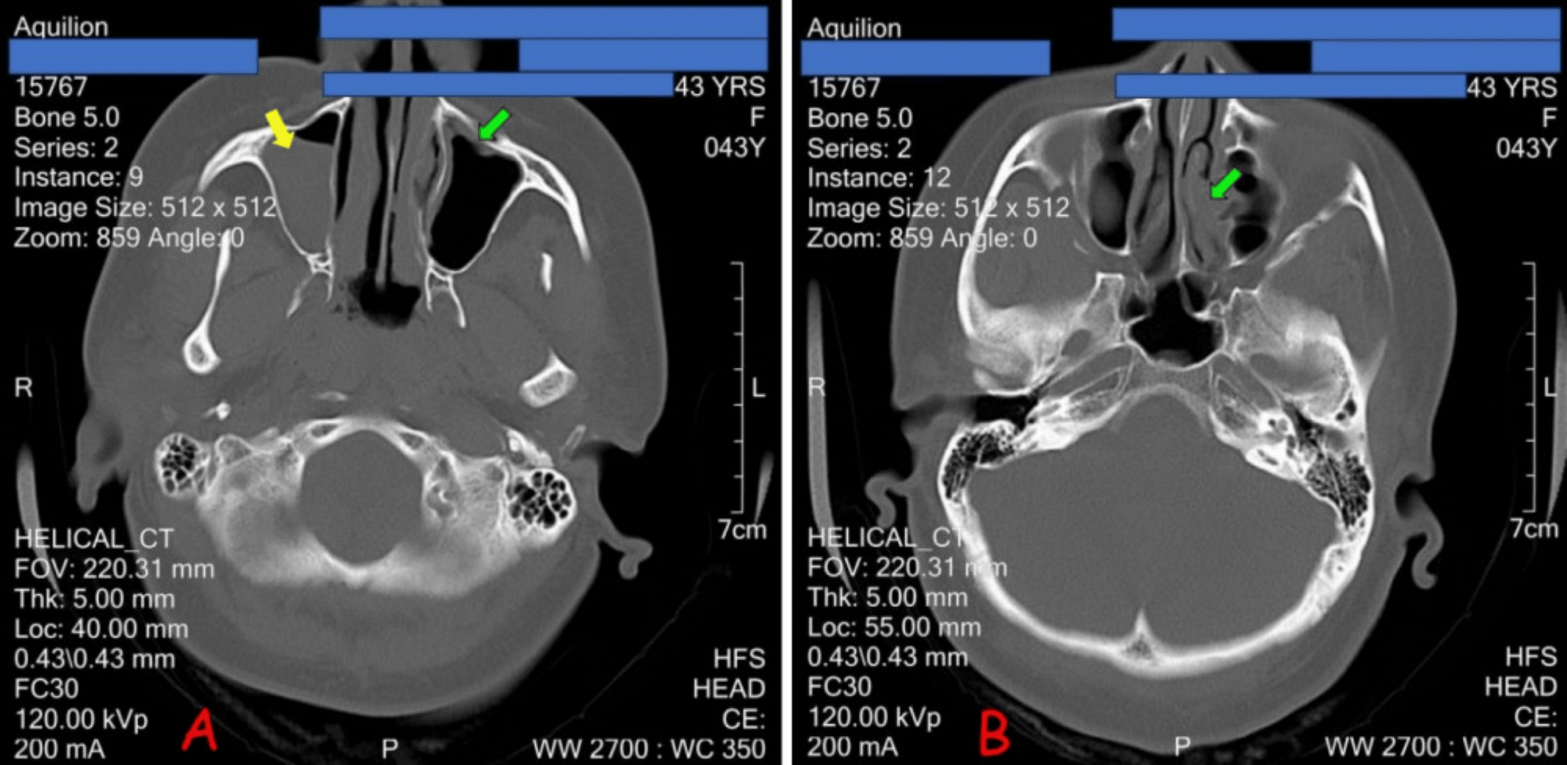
Plain axial CT images of the maxillary sinuses. (A) Isodense mass in the right maxillary sinus (yellow arrow) and increased mucosal thickness in the left maxillary sinus (green arrow). (B) Increased mucosal thickness of the left ethmoidal sinuses (green arrow).
